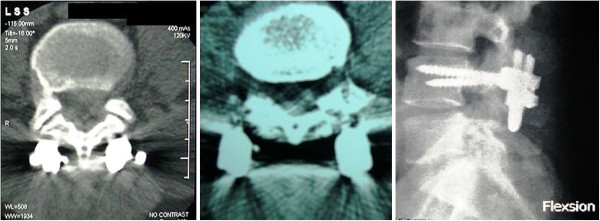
Lumbar midline sagittal and axial T2-weighted magnetic resonance imaging (center, right) showing no degenerated disc, neural compromise, frank instability, or multilevel disease; and extension standing lateral and oblique X-rays (left) showing the pars defect with minimal forward slippage.
